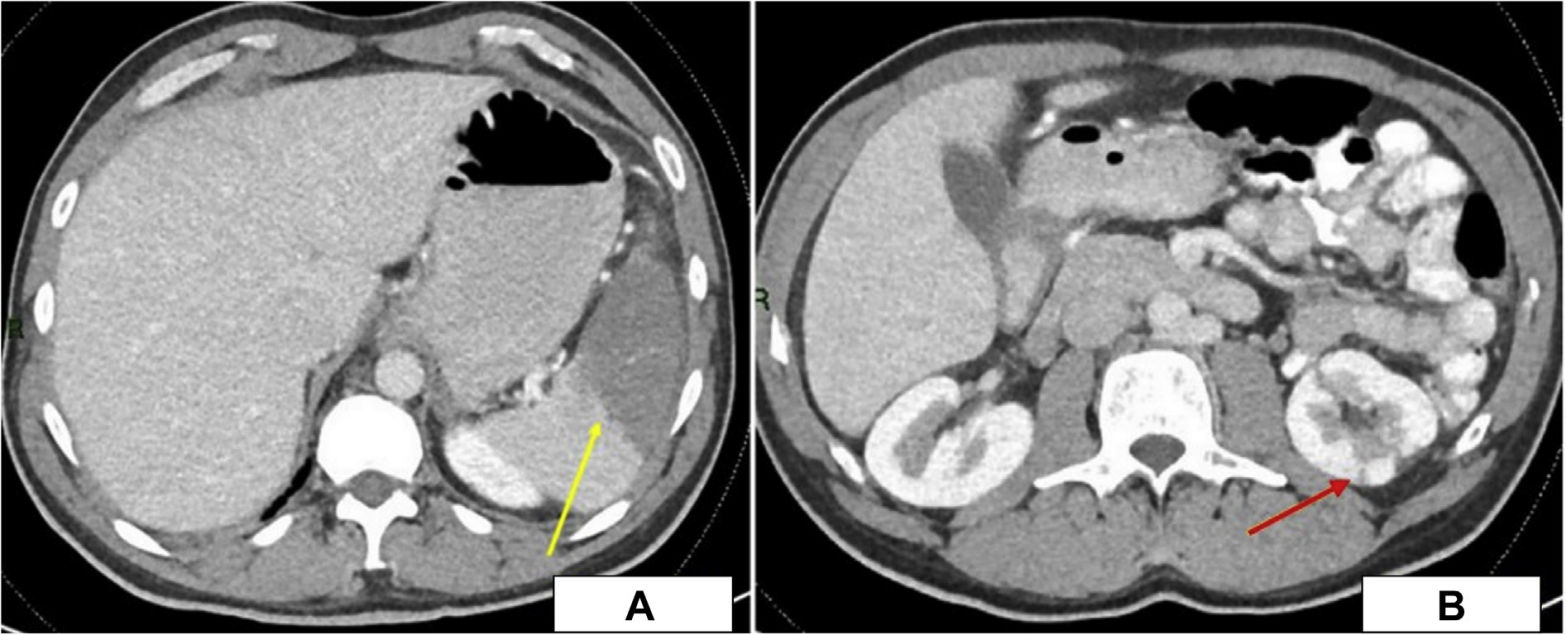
Abdominal computed tomography axial view showing (A) splenic infarct (*yellow arrow*) and (B) left renal infarct (*red arrow*).
